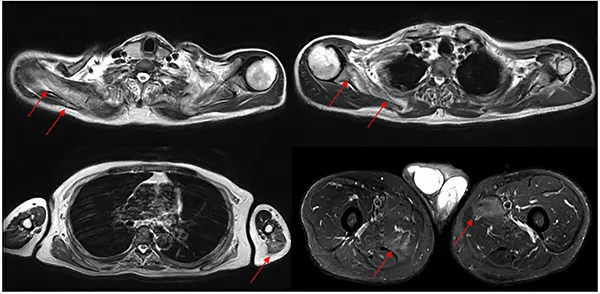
Magnetic resonance imaging (MRI) showed hyperintense areas on STIR images in the right supraspinatus muscle, right subscapularis muscle, left triceps brachii, bilateral obturator externus muscles, right adductor magnus, and left adductor longus. Red arrows indicate hyperintense areas.
